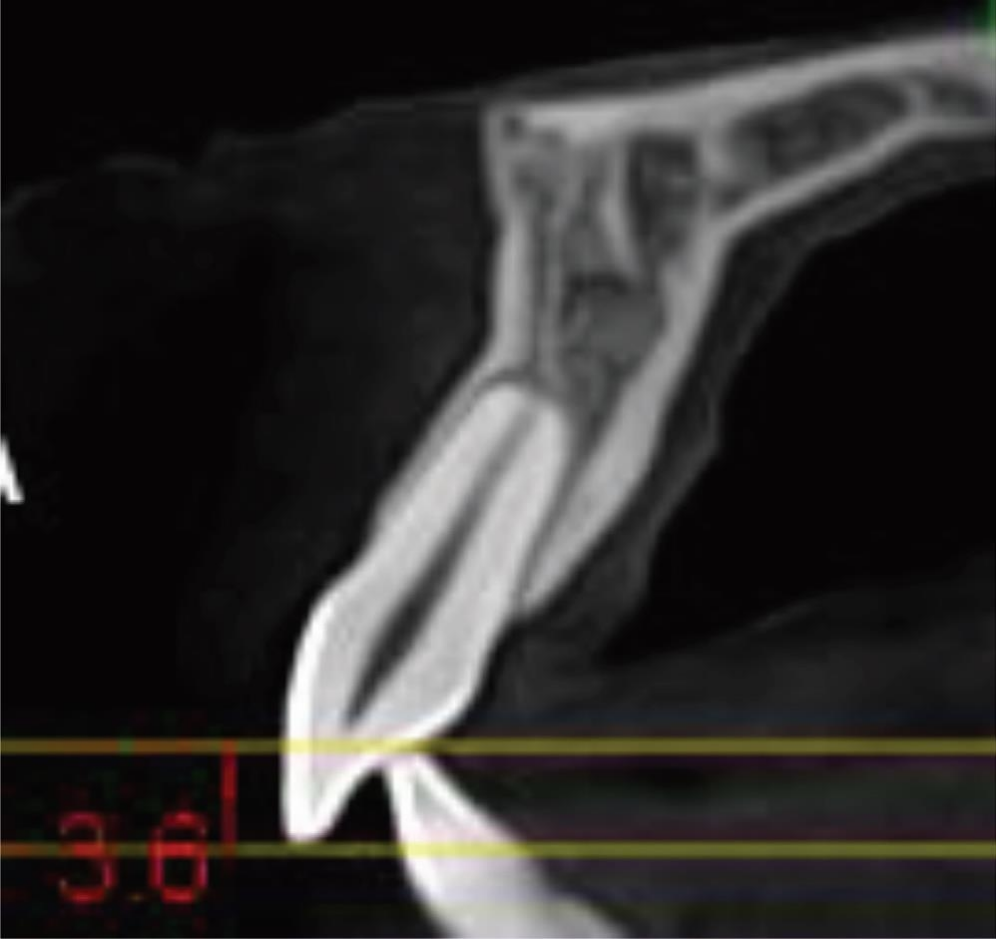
Measurement of overbite in the sagittal view of ULD CBCT radiography.
